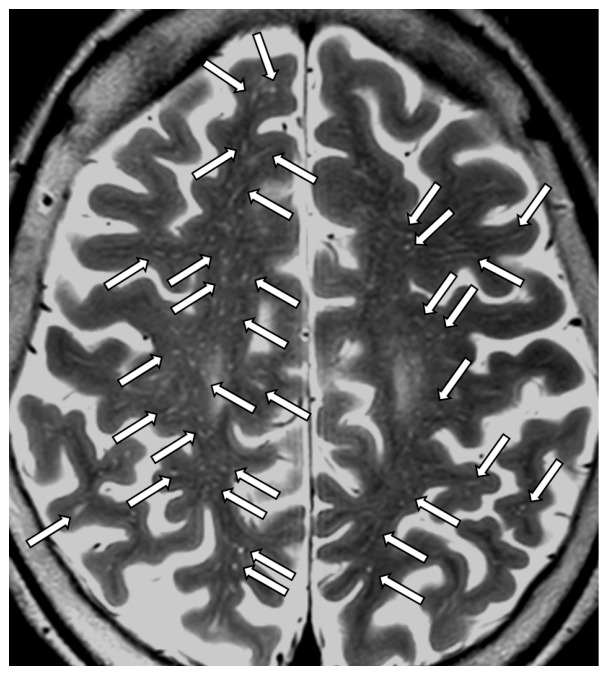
A representative CAA case in an 87-year-old woman (MMSE score, 28). The T2-weighted image shows enlarged CSO-PVS (arrows) in both hemispheres (grade 4).
